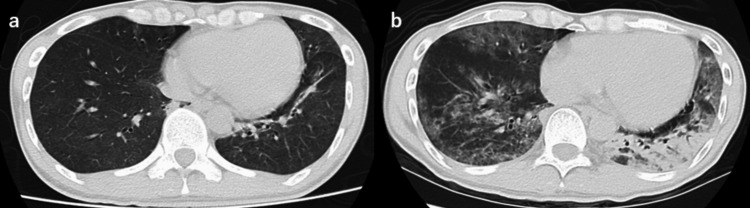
CT scan of the chest. (a) At eight days after the diagnosis of SARS-CoV-2 infection, diffuse ground-glass opacities are observed. (b) At 24 days after the diagnosis of SARS-CoV-2 infection, diffuse ground-glass opacities and infiltrative shadows in the left lower lobe are observed. CT: computed tomography; SARS-CoV-2: severe acute respiratory syndrome coronavirus 2.
